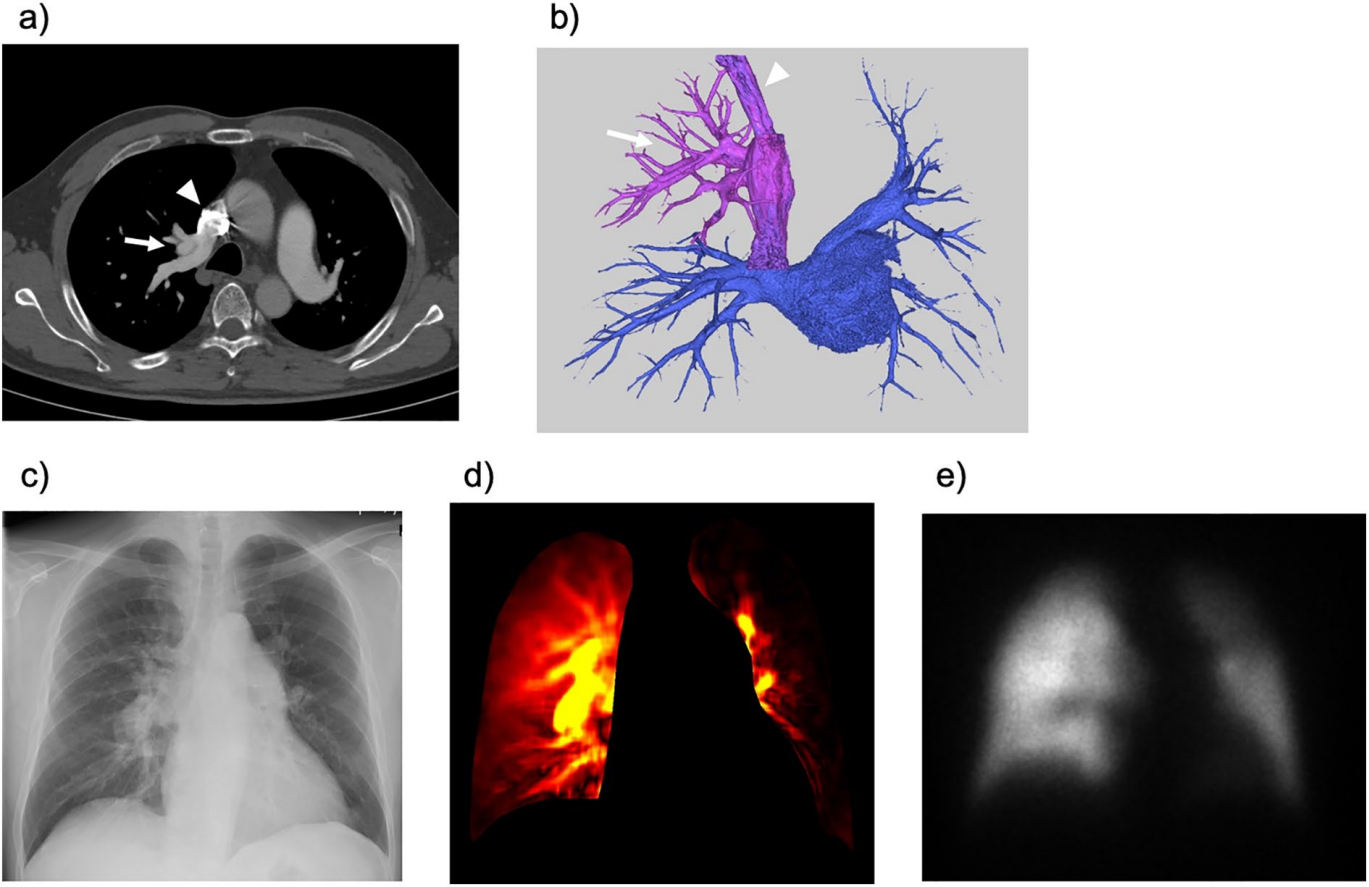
Images of a 50-year-old man with partial anomalous pulmonary venous return (PAPVR). Axial image (a) and 3D image of the pulmonary vein (b) obtained from computed tomography pulmonary angiography showing the right upper pulmonary vein (arrows) draining into the superior vena cava (arrowheads). Chest radiograph showing prominent pulmonary vasculature in the right lung (c). Dynamic chest radiography (d) shows increased pulmonary perfusion in the right lung (right, 85.6%; left, 14.4%), similar to perfusion planar scintigraphy (right 73.8%, left 26.8%) (e)
